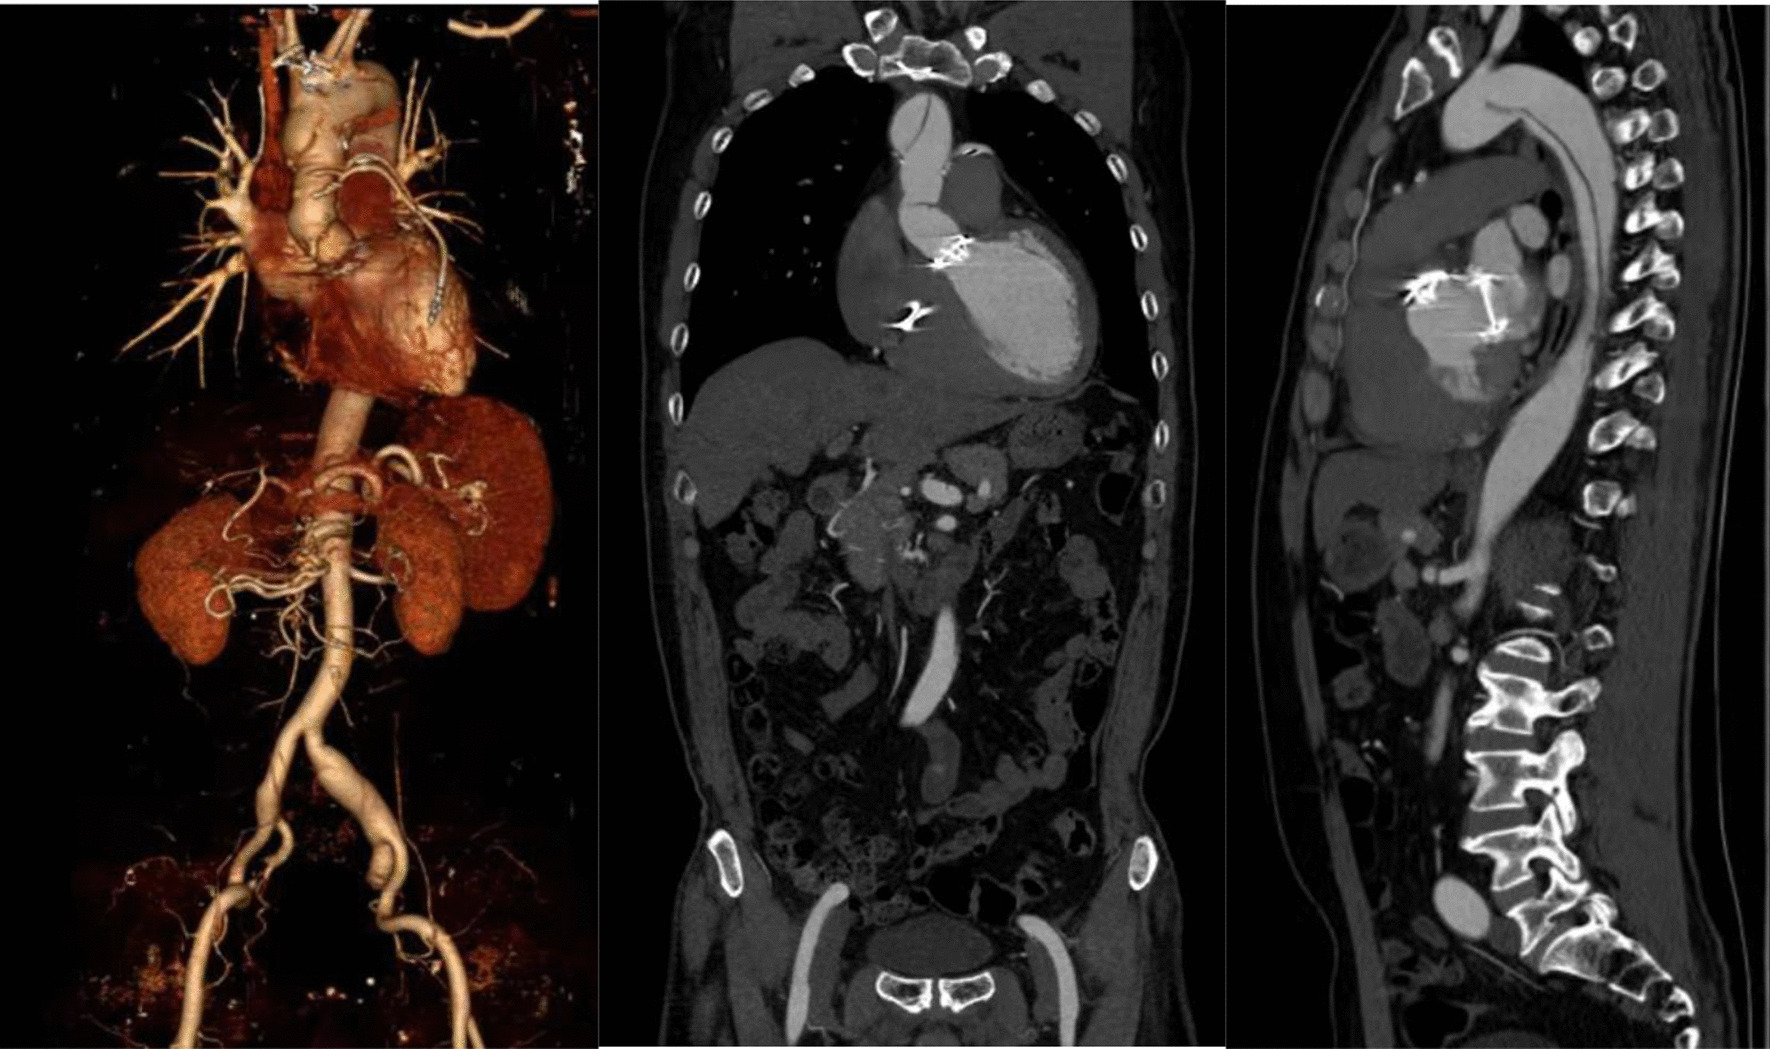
A repeat CT scan of the aorta at the 22ed month after Bentall surgery
